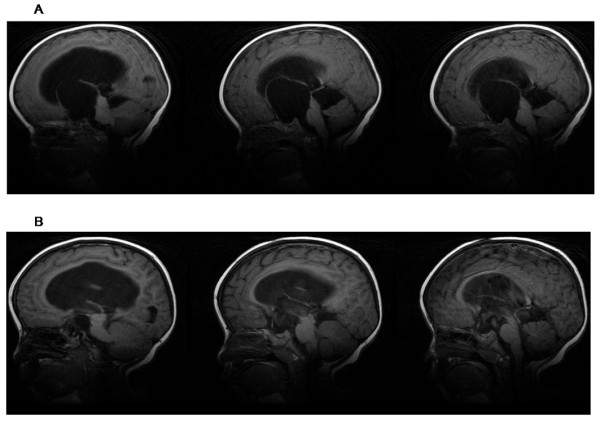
Sagittal magnetic resonance images obtained in same patient. A: Preoperative sagittal T1-weighted image (male, 1 year old) B: Postoperative sagittal T1-weighted image (25 months later).
